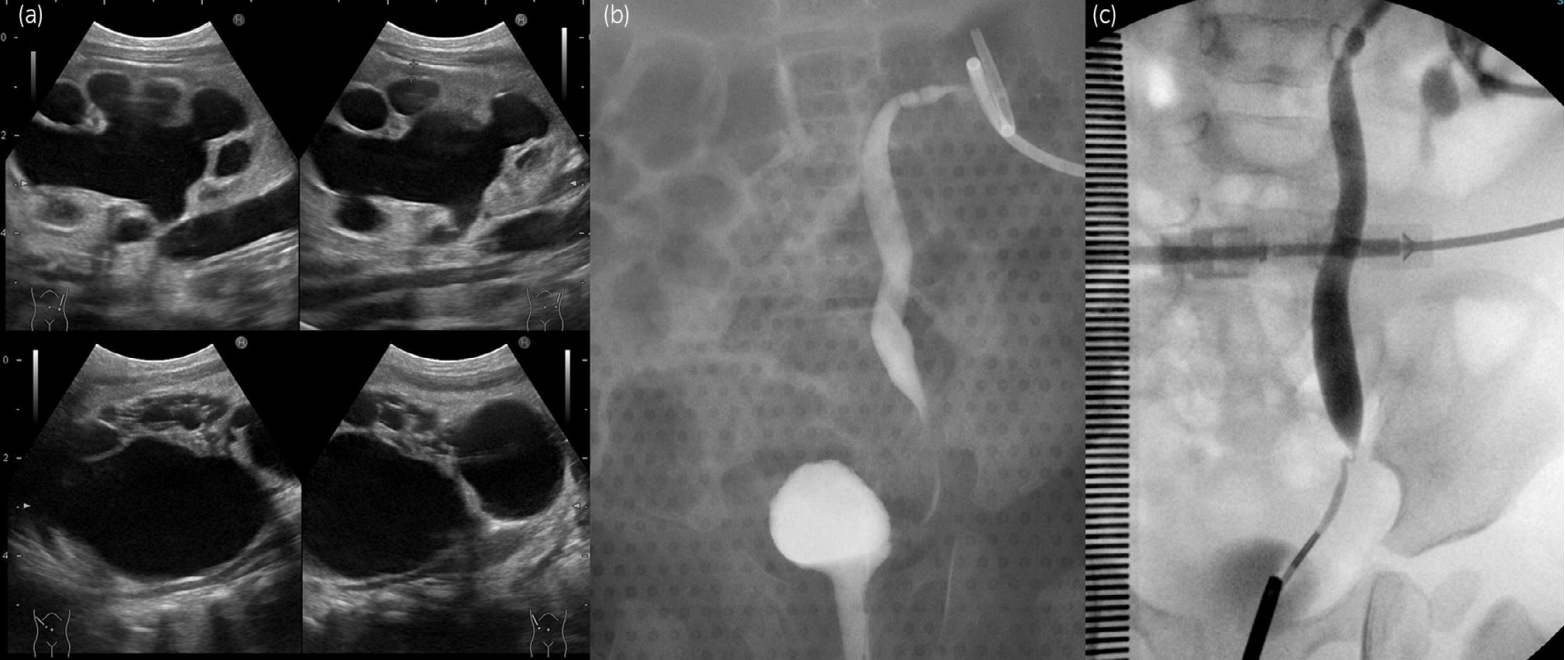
(a) Ultrasonographic images obtained soon after birth showing hydronephrosis grade 4 on the left, left megaureter (upper image), and a right multicystic dysplastic kidney (lower image). (b) A voiding cystourethrogram was obtained at 1 month showing left VUR grade 2 with a very small bladder. (c) Retrograde pyelography was performed at 1 year and 2 months. The site of the stricture was found to be 3 cm cranial from the bladder.
